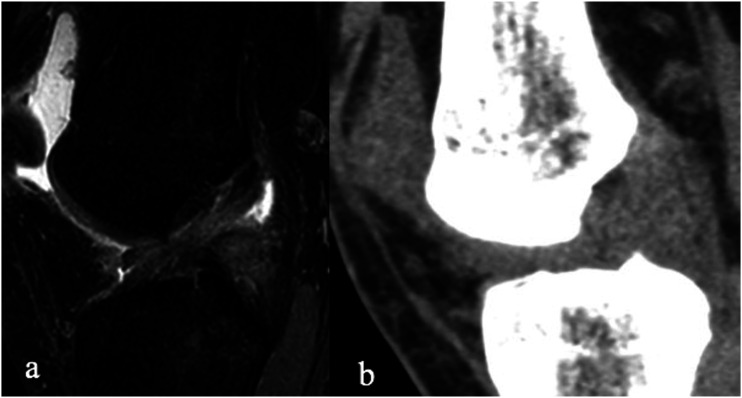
False positive: A 27-year-old male with a normal ACL in arthroscopy that was classified as torn in MRI (Sagittal PD fat sat TE 30 ms., TR 3400 ms., slice thickness 3 mm) (a) and in DECT (b). The Readers were able to freely adjust window settings and do reconstructions in any desired plane when analyzing DECT images.
